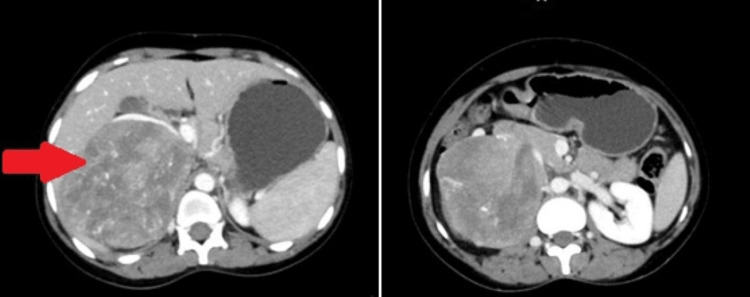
CECT of the abdomen with the red arrow pointing to the large IVC tumor CECT: Contrast-enhanced computed tomography, IVC: Inferior vena cava
